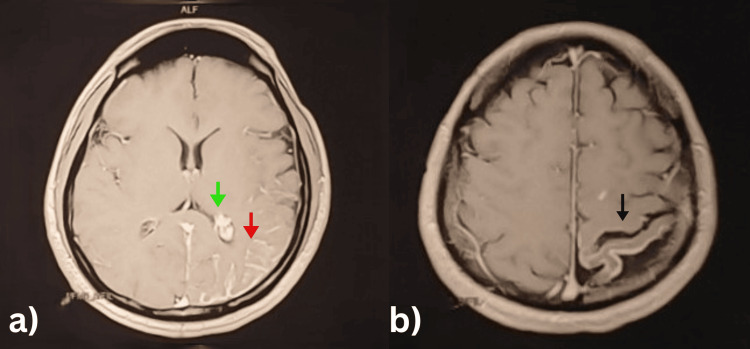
Axial post-contrast T1-weighted MRI Showing (a) enlarged choroid plexus in the lateral ventricle (green arrow) and leptomeningeal enhancement (red arrow), and (b) cortical atrophy (black arrow).
